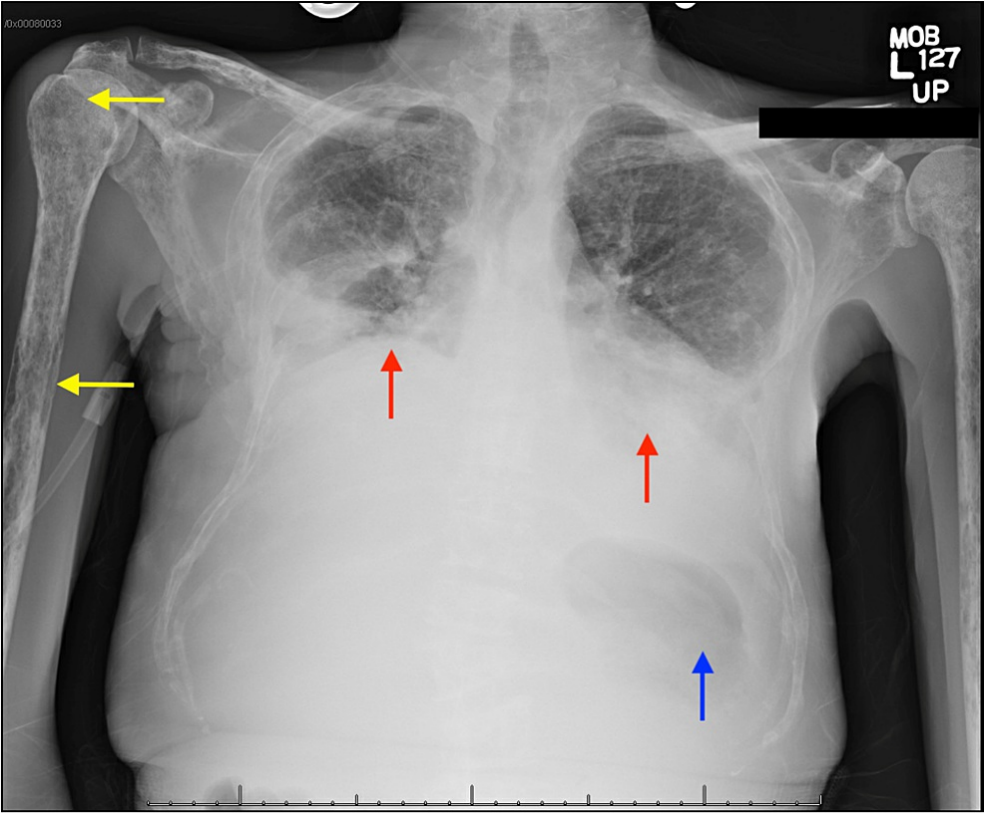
Chest x-ray in an upright antero-posterior view demonstrating bilateral pleural effusions (red arrows), an obscured cardiac silhouette (blue arrow), and diffuse osseous lytic lesions (yellow arrows).
